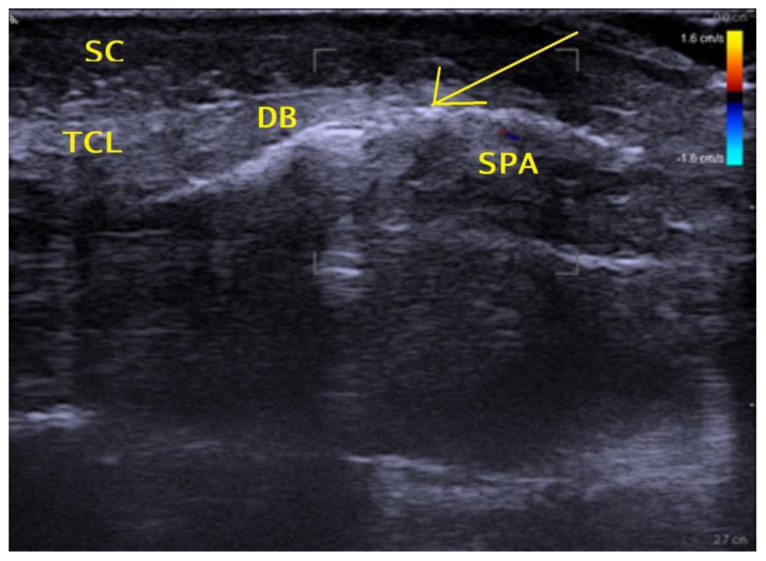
Sagittal distal palmar sonographic view. Arrow = distal needle insertion site; SC = subcutaneous tissue; TCL = transverse carpal ligament; DB = duck’s beak; SPA = superficial palmar arterial arch.
